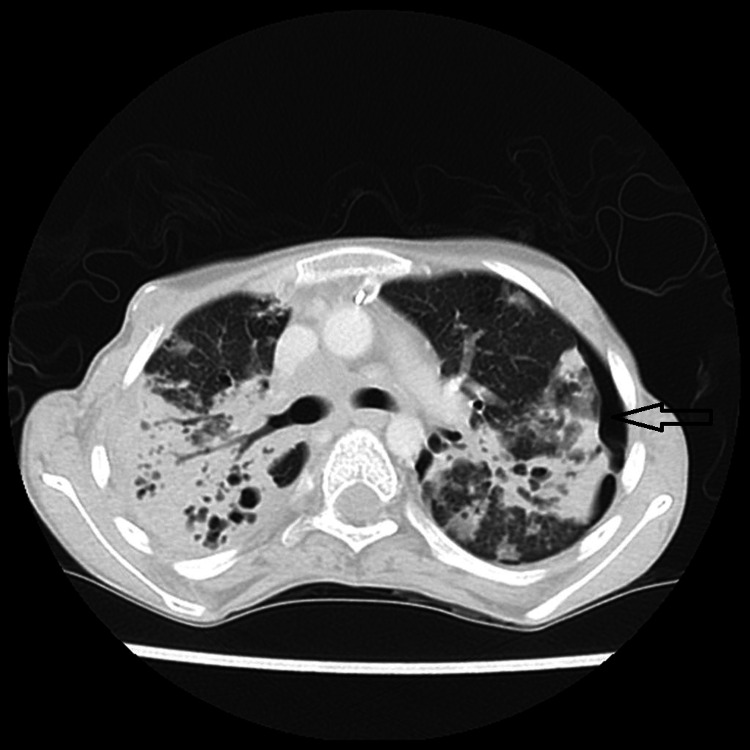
Subtle left-sided pneumothorax (black arrow) not detected on the previous radiograph by both the resident and the attending radiologist
